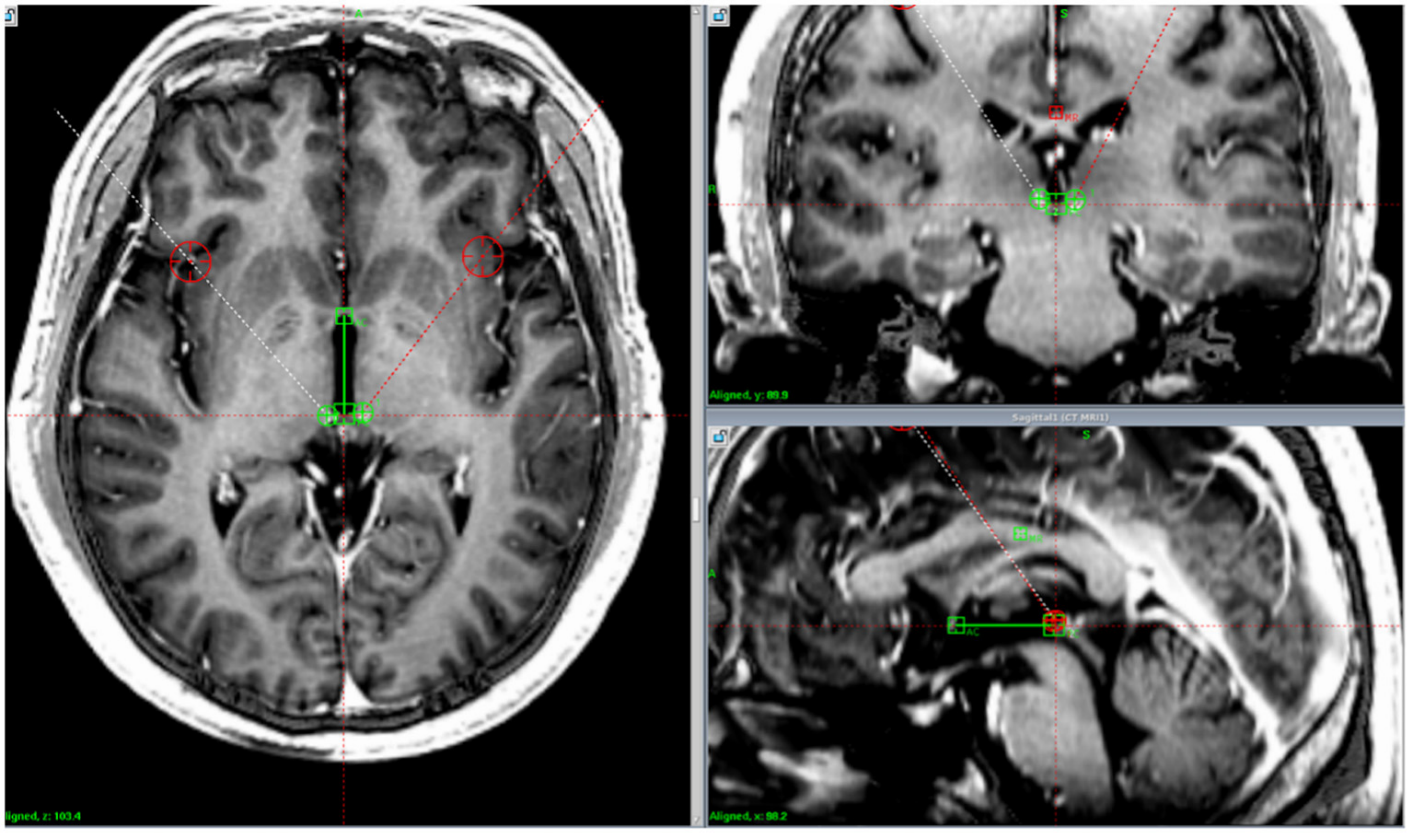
Lead location. Postoperative computed tomography images fused with preoperative magnetic resonance imaging demonstrating the position of the implanted electrodes in the habenula in one patient.
